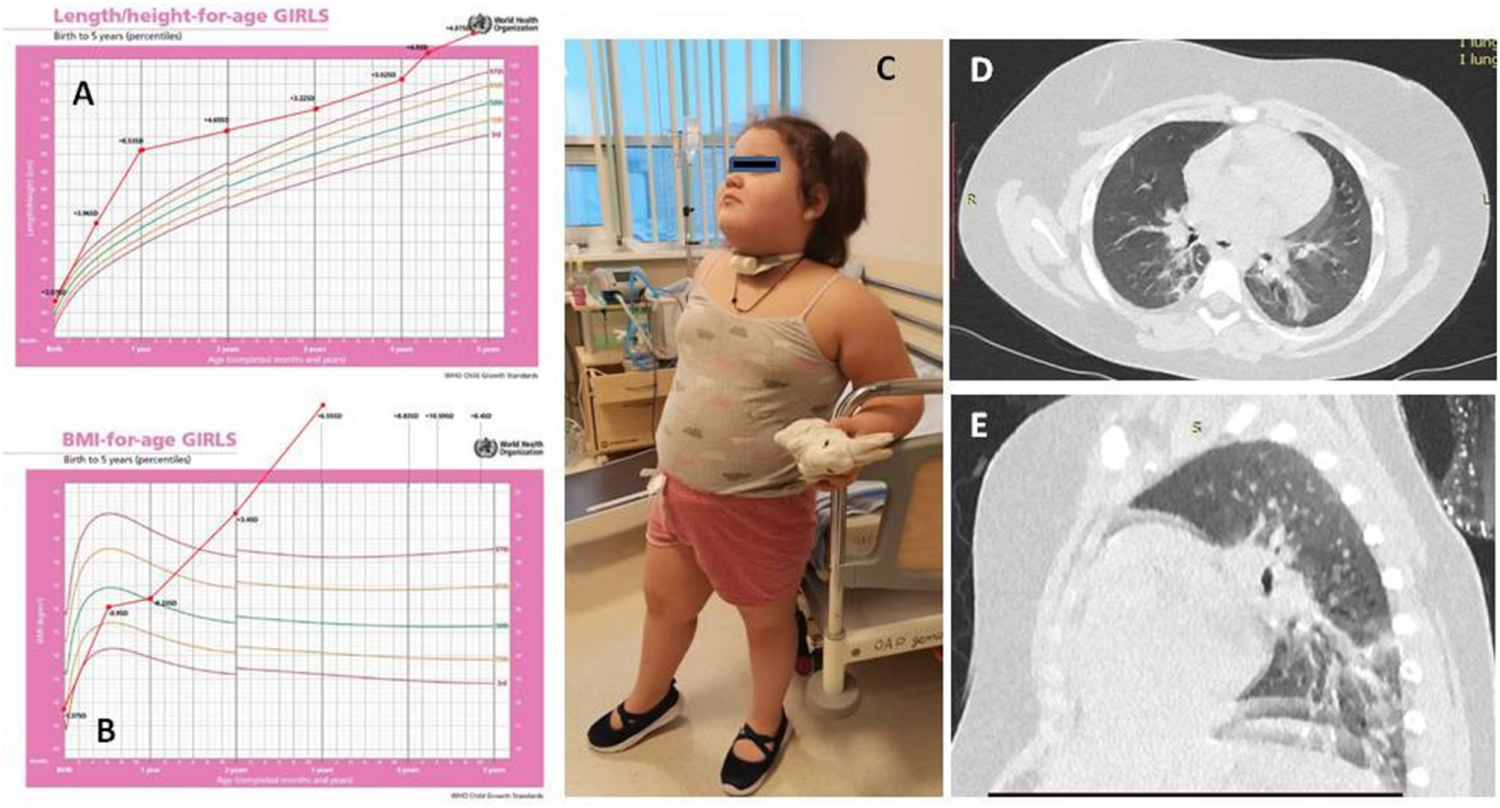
The dynamics of height (A) and body mass index (B) of the patient. Patient's picture (C). Chest CT (D,E).
